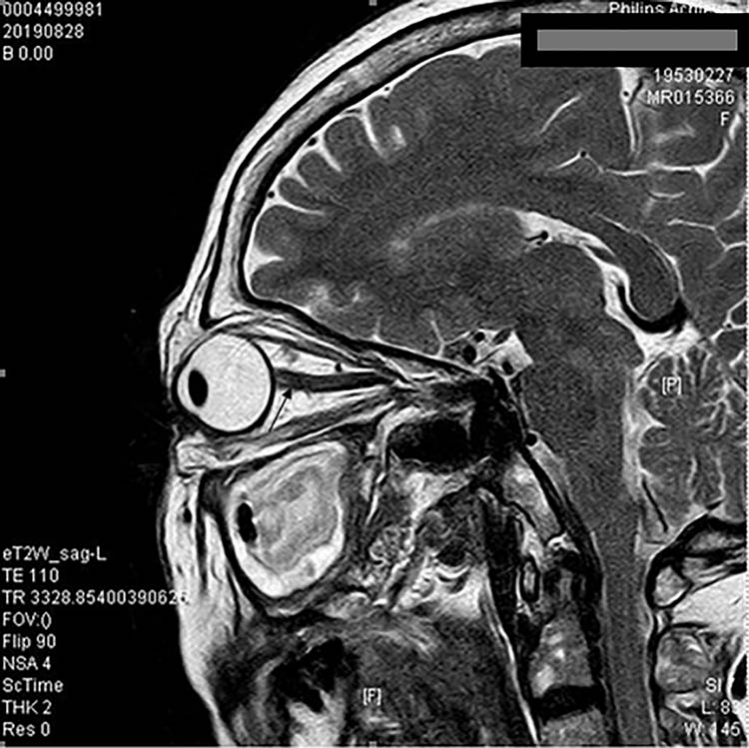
Orbital MRI showed local edema of the right optic nerve.
